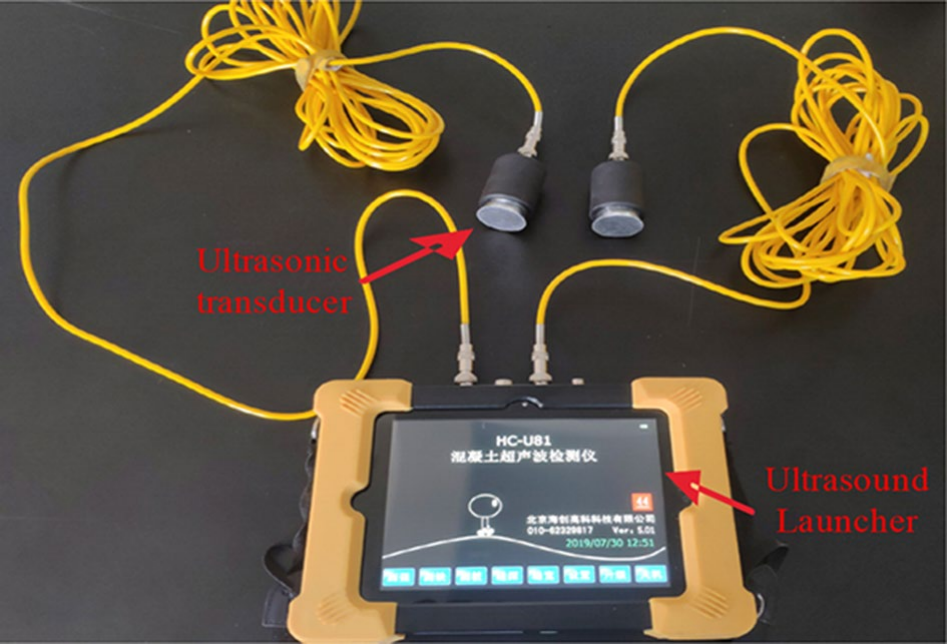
Ultrasonic wave velocity testing system used in this study.
